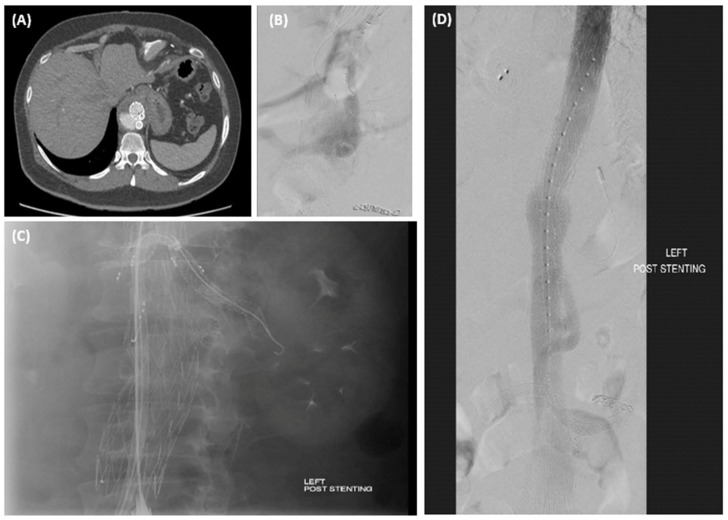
(A) Endoleak observed on CT. (B) Selective branch cannulation for diagnosis of Type 3 Endoleak. (C) Use of steerable sheath for cannulation and stent extension of branches. (D) Resolution post-stenting.
